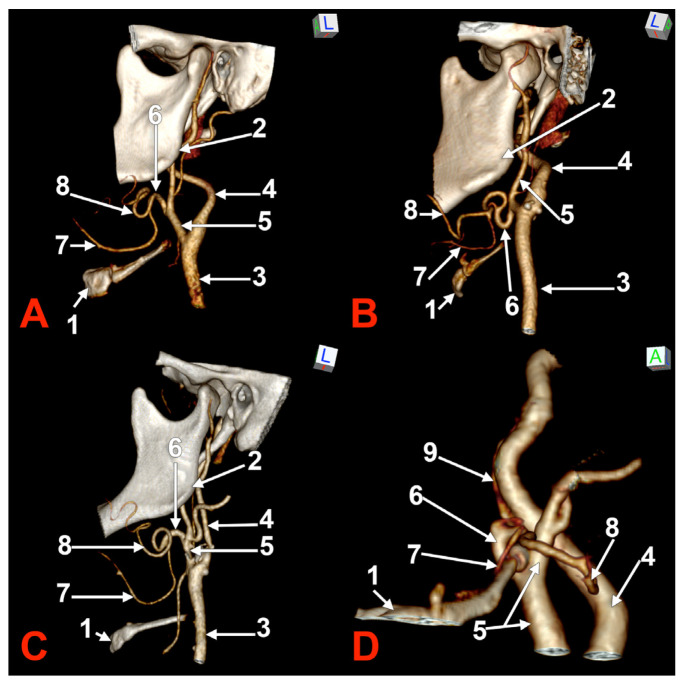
Morphological variants of the left unilateral linguofacial trunk. Computed tomography angiograms. Three-dimensional volume renderings. Infero-lateral (A–C) and antero-lateral (D) views. 1. hyoid body; 2. gonial angle; 3. common carotid artery; 4. internal carotid artery; 5. external carotid artery; 6. linguofacial trunk; 7. lingual artery; 8. facial artery; and 9. ascending pharyngeal artery.
